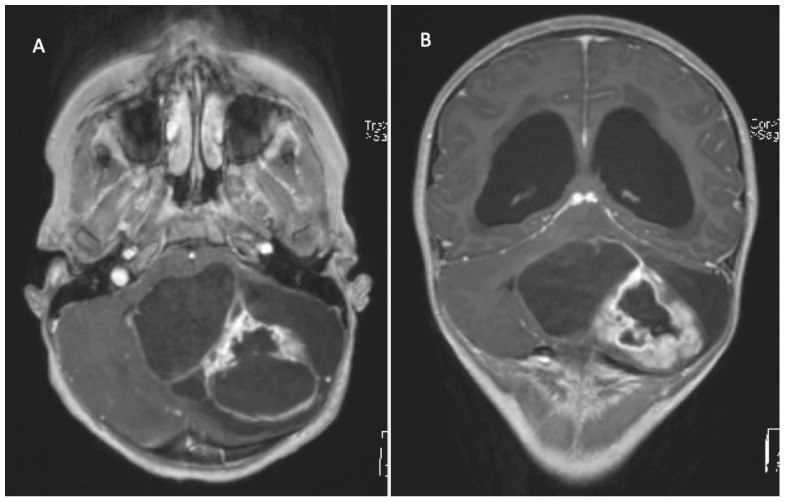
12 year old girl with acute signs of raised ICP and schooling difficulties. Contrasted T1 MRI shows large cystic tumor with contrasting solid components and chronic hydrocephalus (A,B). Diagnosis: pilocytic astrocytoma (WHO grade 1).
